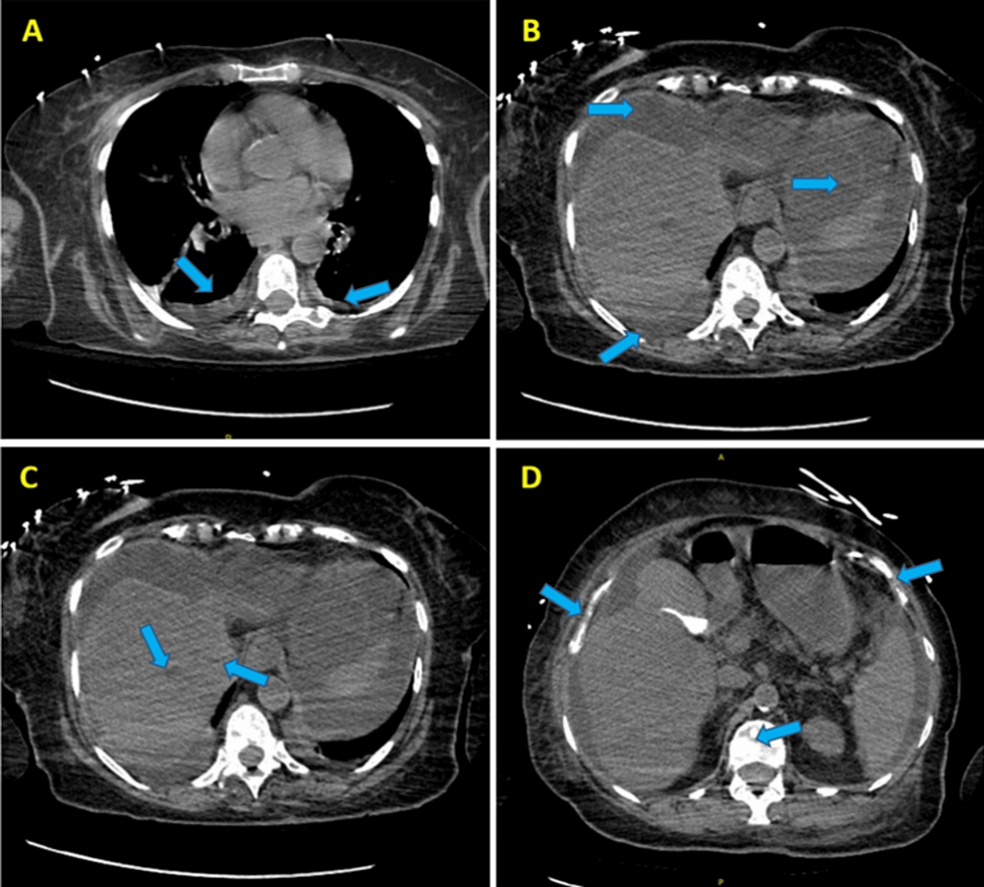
Computed tomography (CT) scan of the abdomen and pelvis without intravenous contrast. (A) The axial view shows small fluid collections around the lungs, likely indicating bilateral pleural effusions at the lung bases, marked by blue arrows. (B) The axial view reveals a moderate-to-large amount of free fluid in the abdomen (peritoneal cavity), highlighted by blue arrows. (C) The axial view displays multiple hepatic lesions pointed out by blue arrows, likely indicative of metastatic disease, but their characterization is uncertain without contrast, unlike the previous contrast-enhanced exam. (D) The axial view presents multiple areas of increased bone density (sclerosis) throughout the skeleton, identified by blue arrows, suggesting metastatic disease. The scan was conducted without intravenous contrast due to the patient's renal function. While this scan supplies valuable information, contrast enhancement could provide a more precise assessment of blood flow and help identify lesions, such as iso-dense lesions, which may be challenging to detect on a non-contrast scan.
